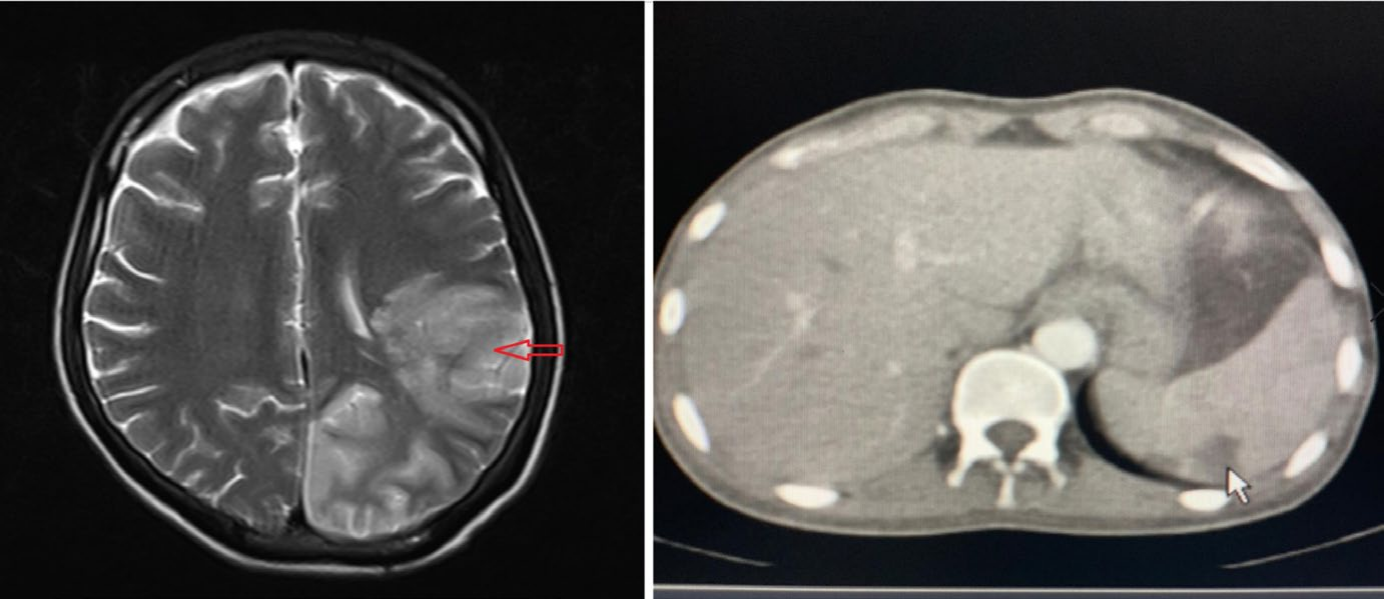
Left panel: Brain MRI showed hyperintense signals in parietal (red arrow) and occipital regions in T2 sequence in favor of infarction. Right panel: Abdominal CT showed hypodense lesions in lower pole of spleen (white arrow) suggestive of infarction
